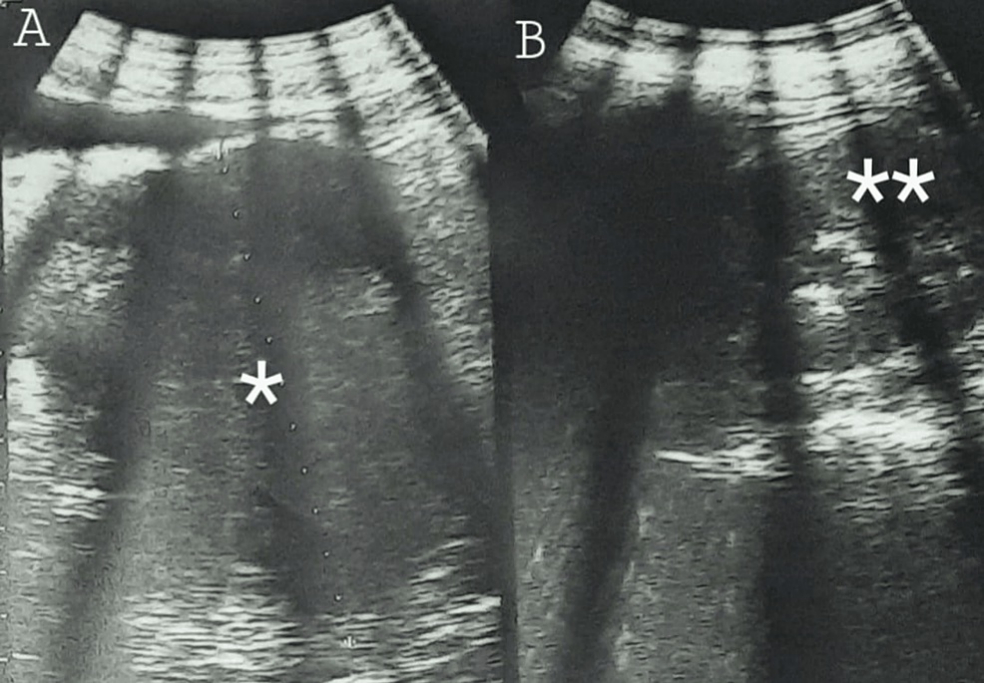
Abdominal-pelvic ultrasound images A: A solid hypoechoic pelvic mass (*). B: A left hydronephrosis ()
